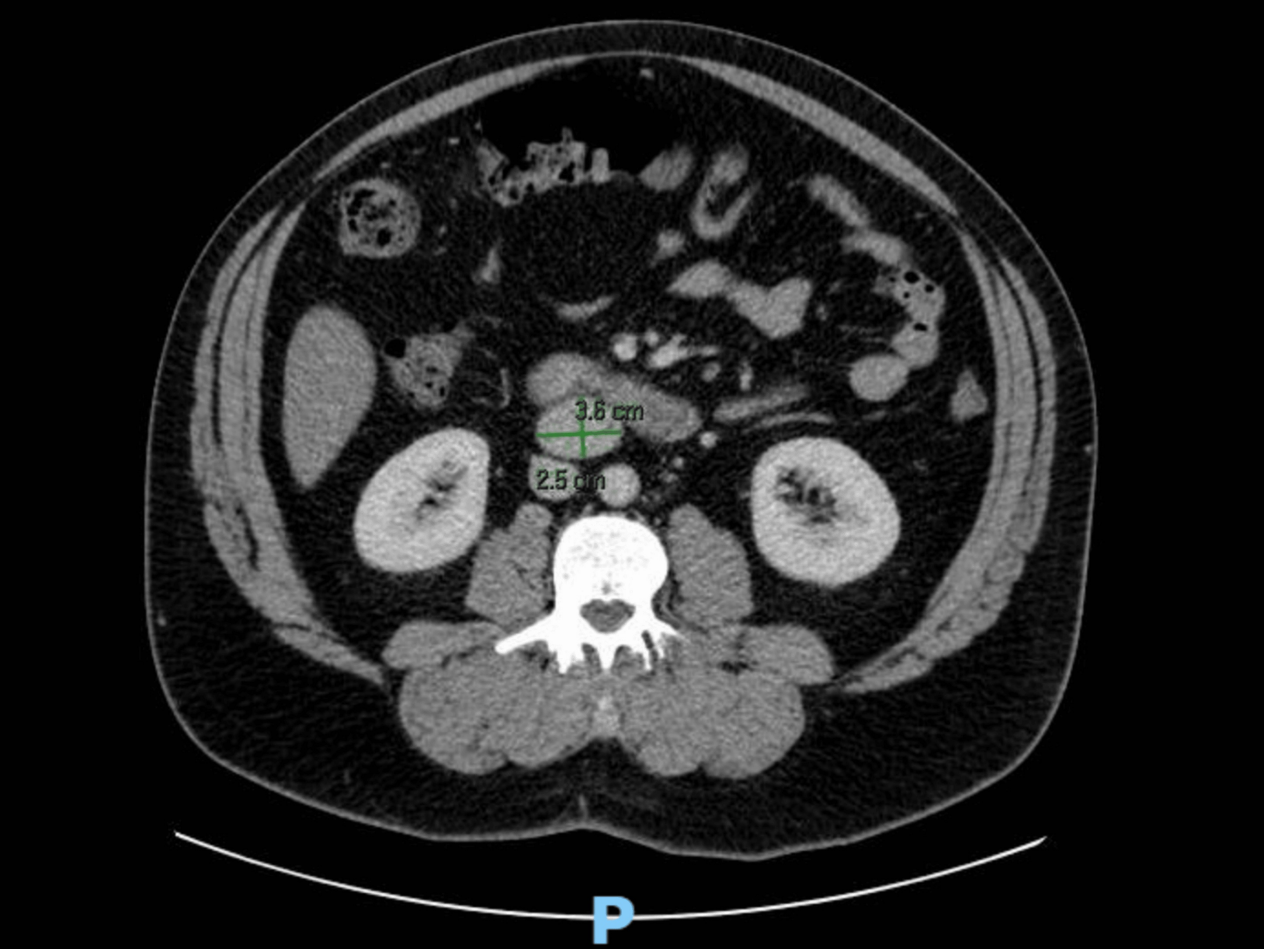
CT abdomen pelvis with IV contrast. Axial view. The image shows a 3.6 cm measurement transverse and 2.5 cm anteroposterior.
